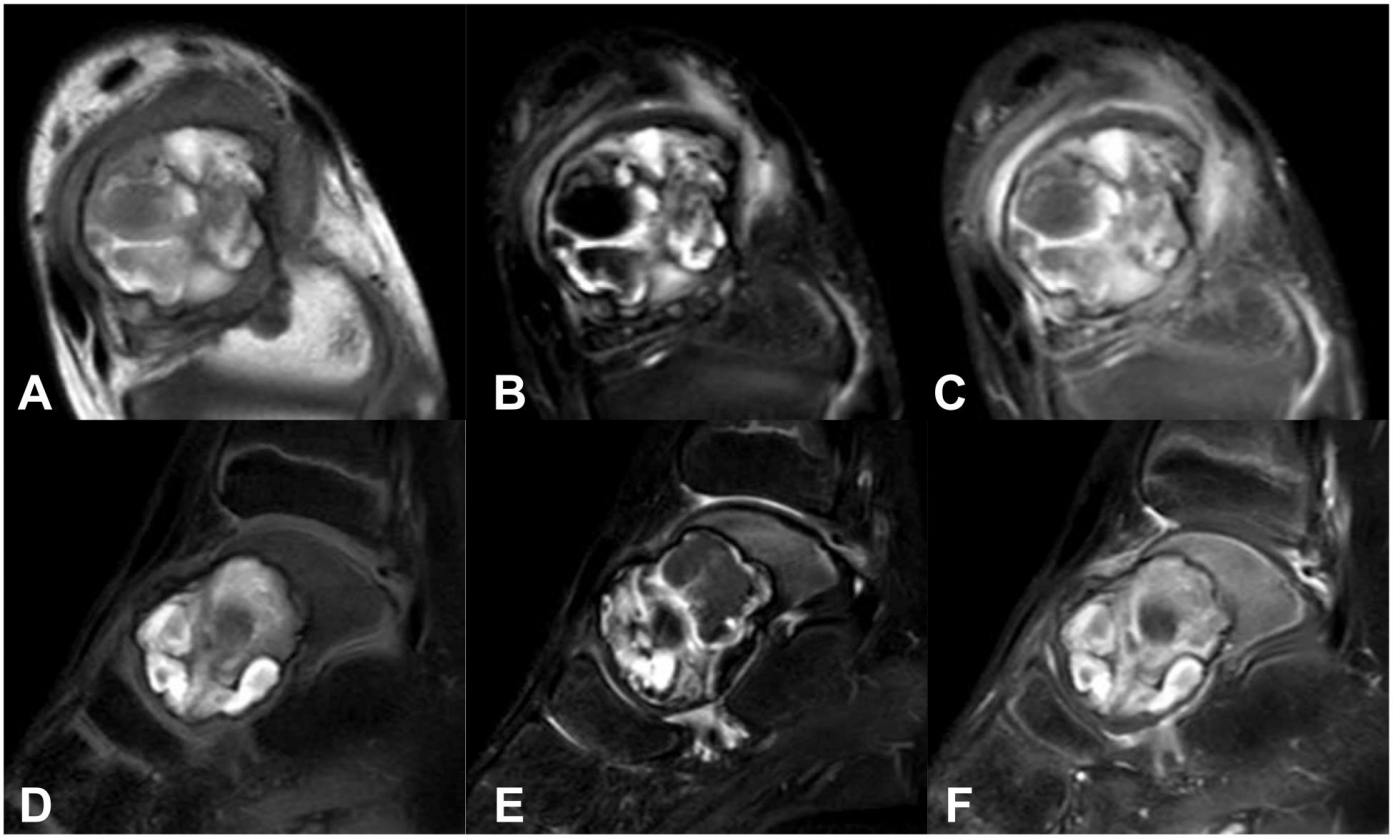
In the MRI examination, T1: Fat suppression revealed high signal, T2: Fat suppression revealed a mixture of low and high signals suggesting the presence of bleeding, and fat suppression on gadolinium contrast enhanced the peripheral area slightly ((A) T1 axial, (B) T2 fat suppression axial, (C) fat suppression on gadolinium contrast-enhanced axial, (D) T1 fat suppression sagittal, (E) T2 fat suppression sagittal, and (F) fat suppression on gadolinium-contrast-enhanced sagittal).
